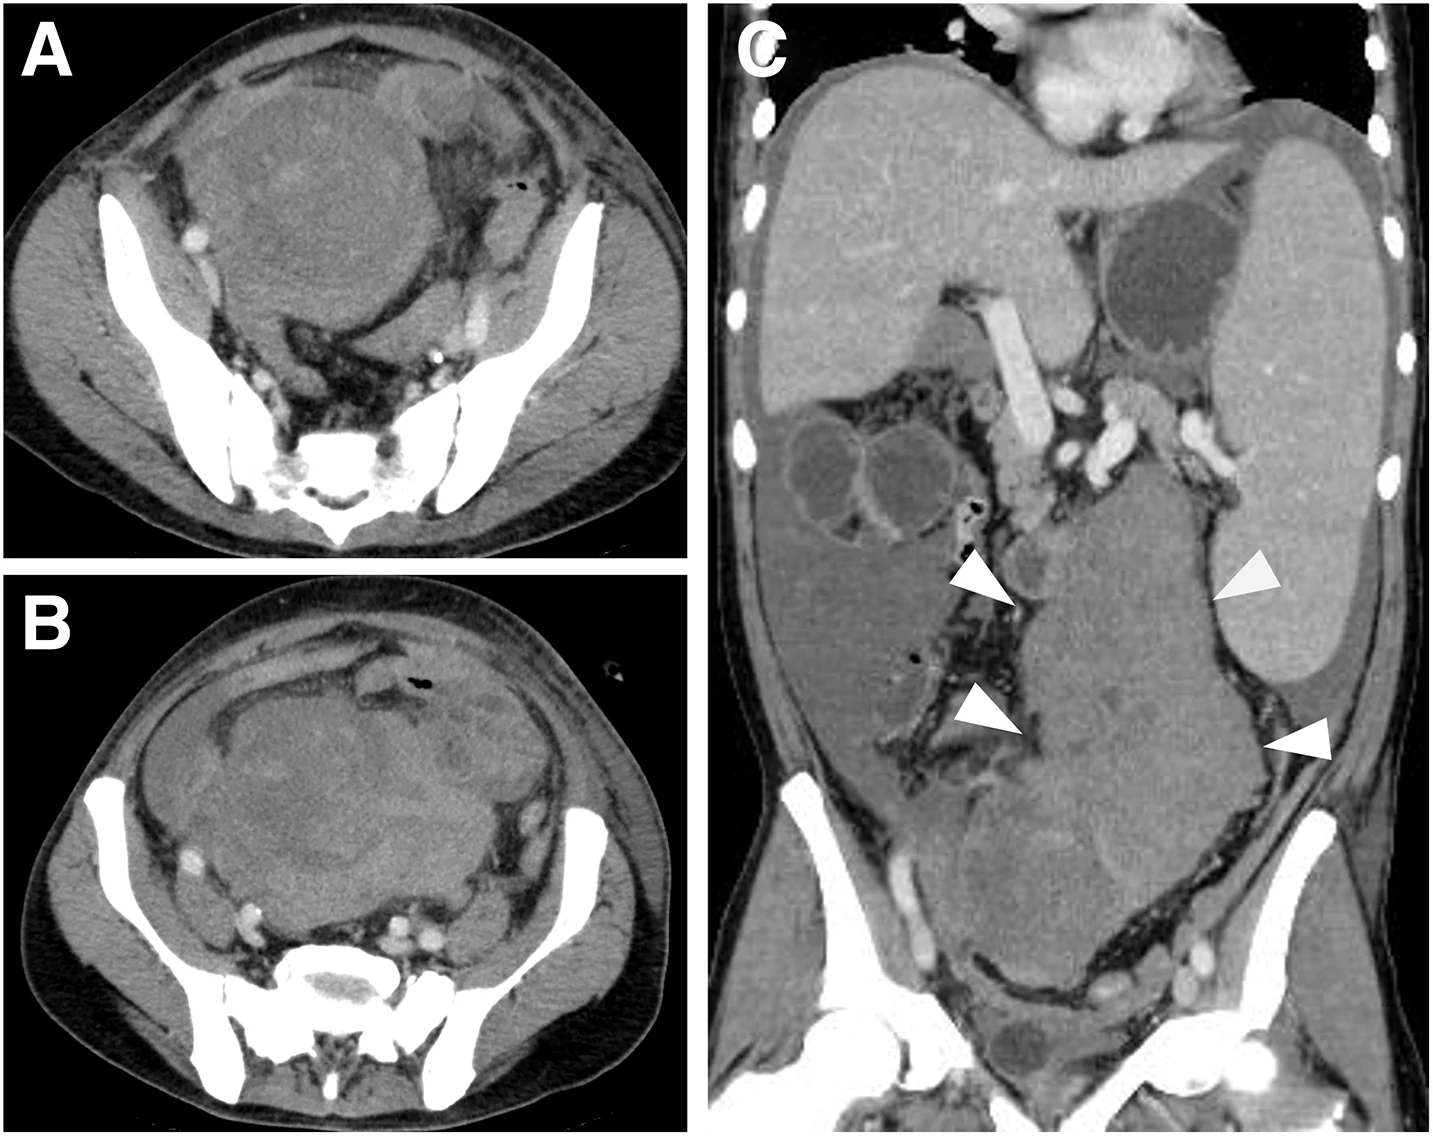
Preoperative contrast-enhanced CT findings. (A) Axial image showing a 9-cm pelvic mass with poor enhancement. (B) Axial image demonstrating focal wall disruption of the pelvic mass with surrounding hyperdense hematoma. (C) Coronal image indicating rupture of the pelvic mass, surrounded by hyperdense hematoma (arrowheads) and hemoperitoneum.
